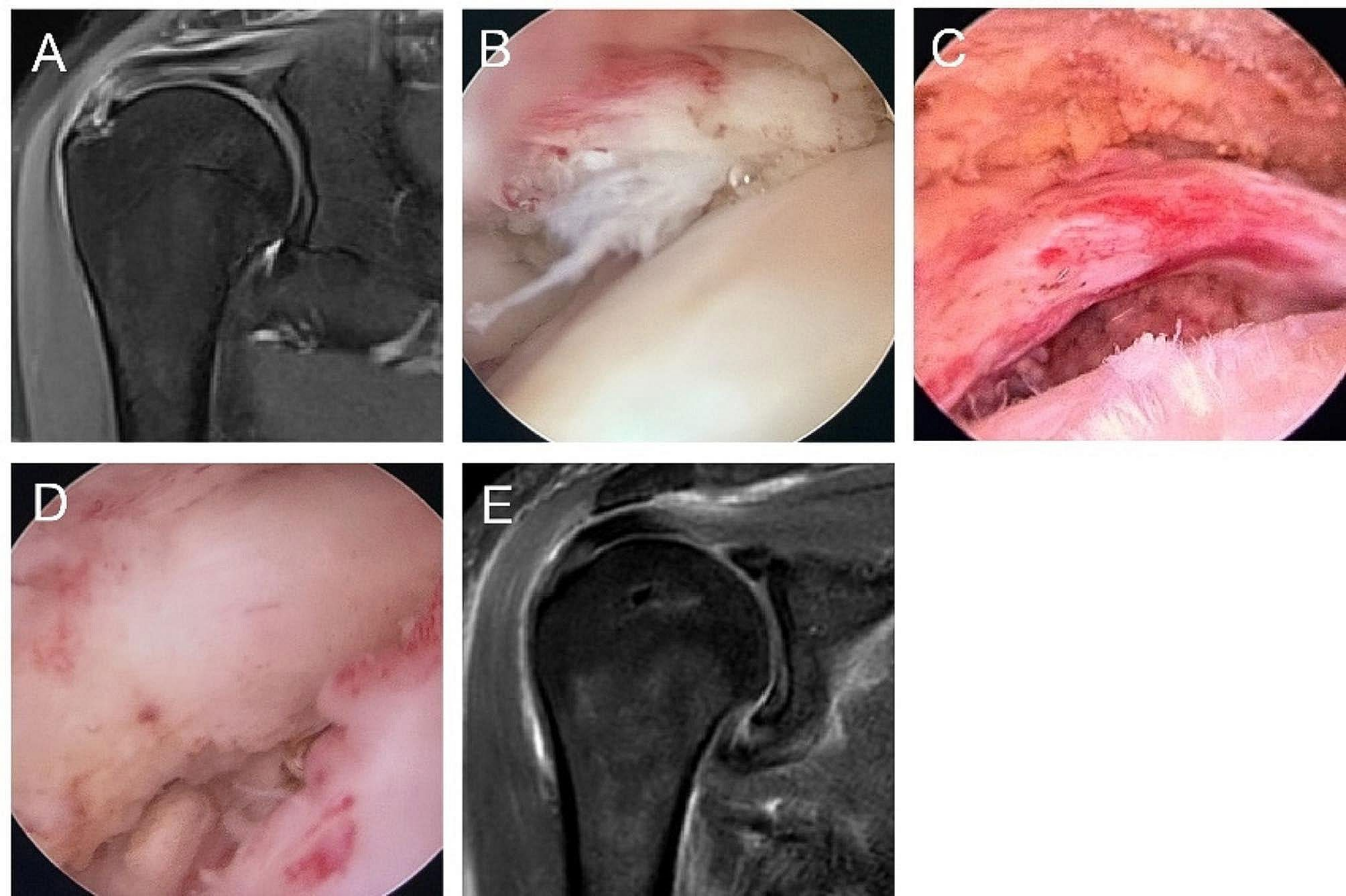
The patient underwent the arthroscopic full-thickness repair method (A) Preoperative magnetic resonance imaging showed Ellman III partial articular-sided supraspinatus tendon tear; (B) Partial tear of the supraspinatus tendon at the articular side in the glenohumeral joint; (C) Partial injury of supraspinatus tendon transforms into a full-thickness injury; (D) The supraspinatus tendon was effectively repaired within the glenohumeral joint; (E) Postoperative magnetic resonance imaging indicated satisfactory repair of the rotator cuff
